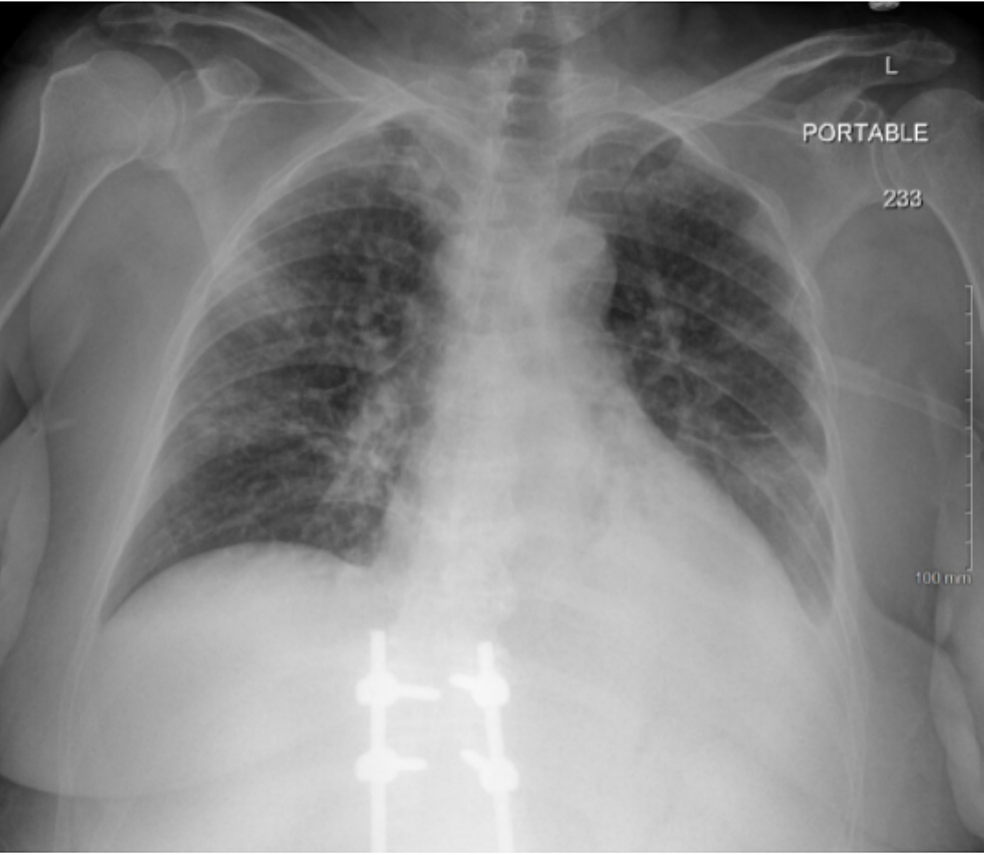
Chest X-ray on presentation to tertiary care center.
